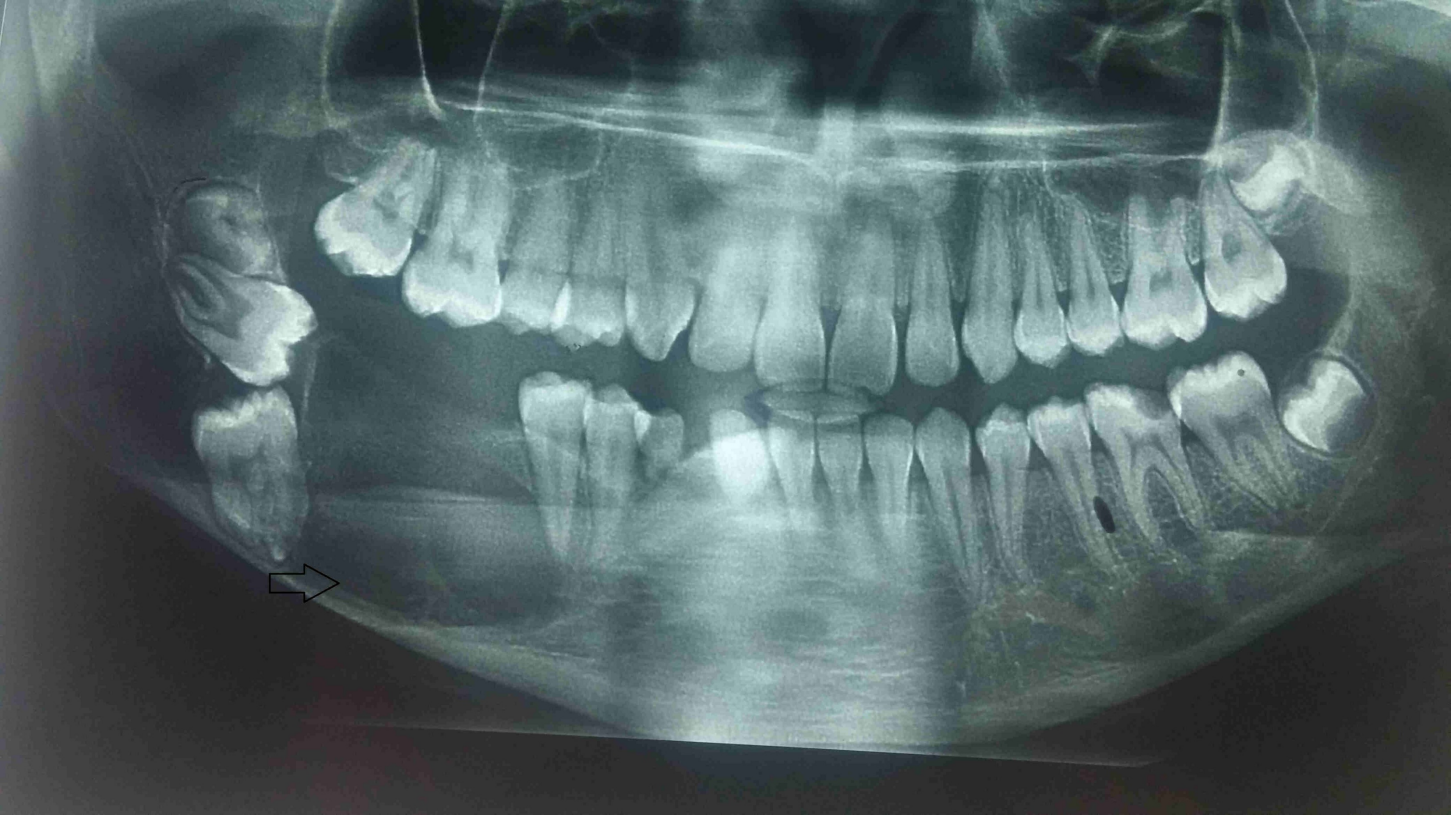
Orthopantomogram showing a well-defined unilocular radiolucent lesion extending from 44 to the retromolar area displacing and impacting 46, 47. The alveolar bone is destroyed in the 46 to 48 area, with intact lower border (Arrow).
